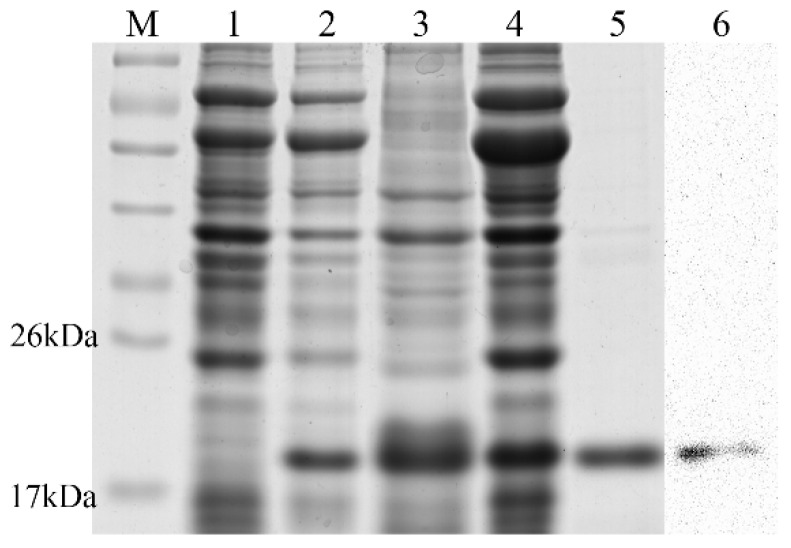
Analysis of the expressed protein via SDS-PAGE. M: protein marker, Lane 1: total proteins from *E. coli* pG-KJE8/BL21 containing recombinant plasmid before IPTG induction, Lane 2: total proteins from *E. coli* pG-KJE8/BL21 containing recombinant plasmid after IPTG induction, Lane 3: inclusion body after ultrasonication, Lane 4: supernatant after ultrasonication, Lane 5: recombinant protein purified using Ni^2+^-NTA column, Lane 6: Western blot of recombinant protein.
